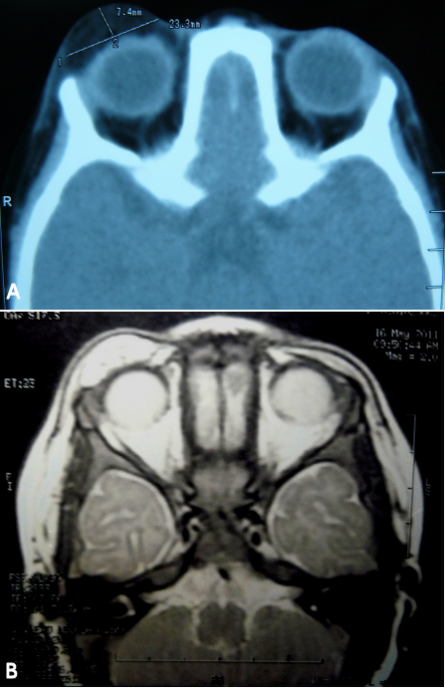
CT images show a 7.4×23.3 mm-sized low density mass in the upper eyelid of the right orbit (A). T2-weighted magnetic resonance imaging (MRI) presenting a high signal intensity (B).
